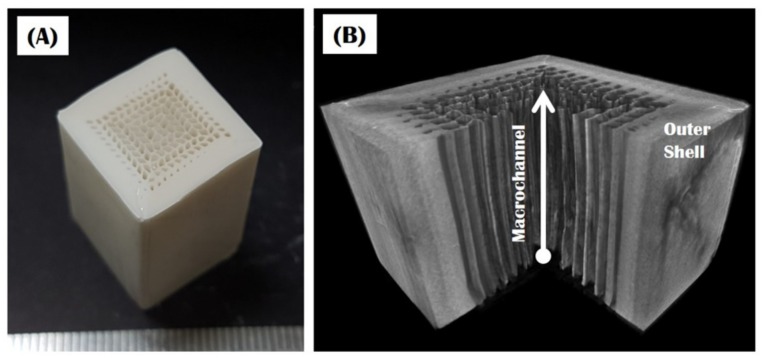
(A) Optical image and (B) reconstructed μ-CT image of the bioinspired CaP scaffold, showing the external and internal macroporous structures.
